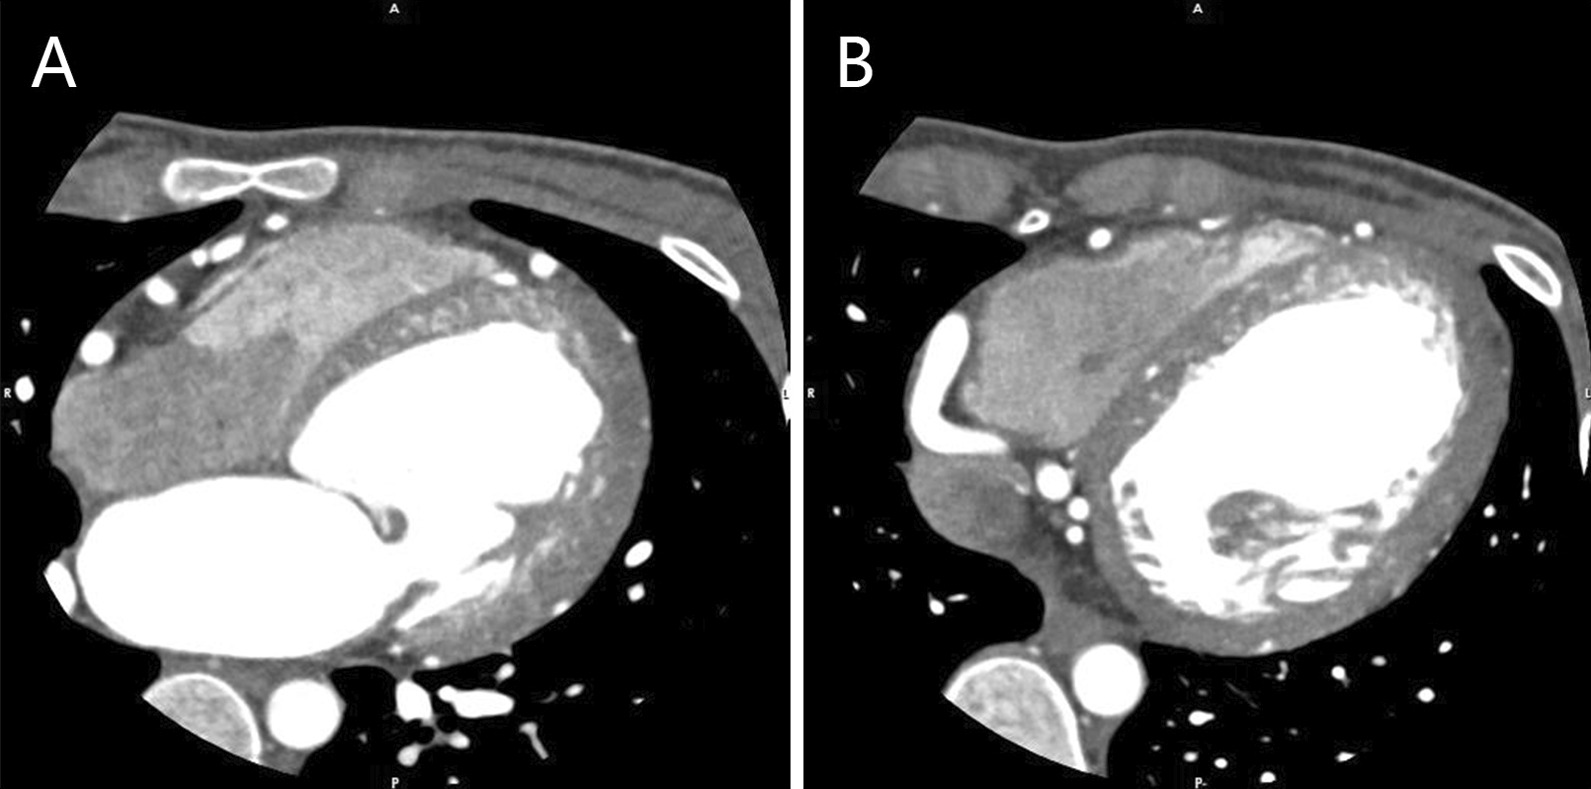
Enhanced computed tomography of the small anterolateral papillary muscle (a) and the normal posteromedial papillary muscle (b)
